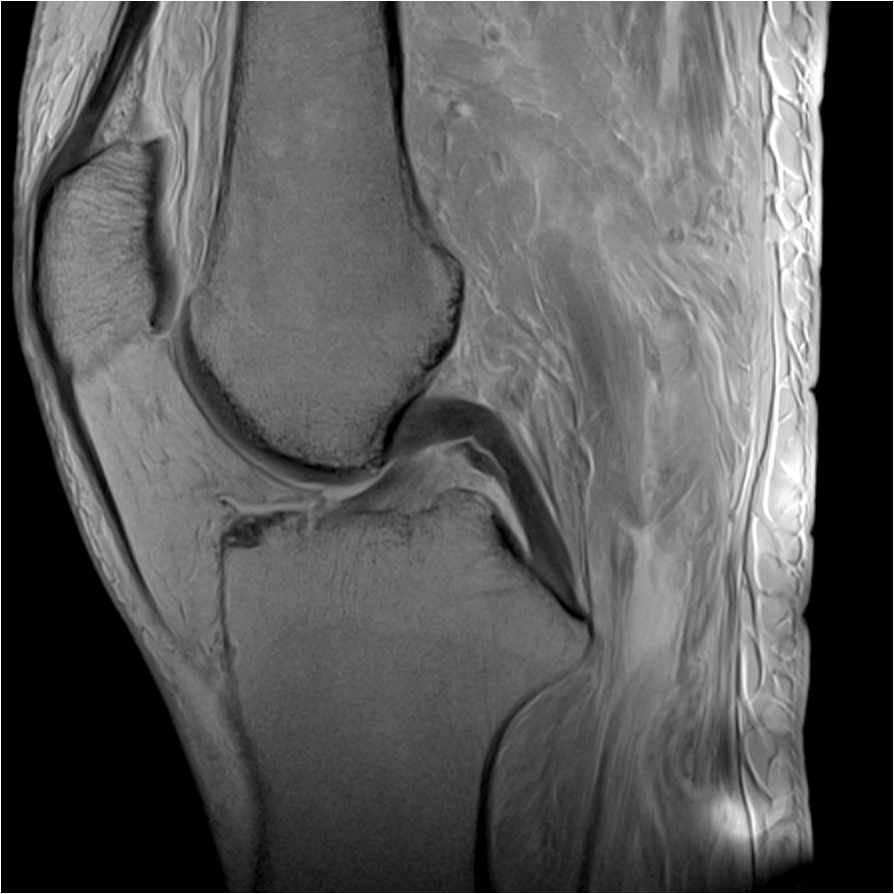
Very good quality of sagittal MRI image of the knee joint, skeletal and soft tissue structures are easily identifiable.
